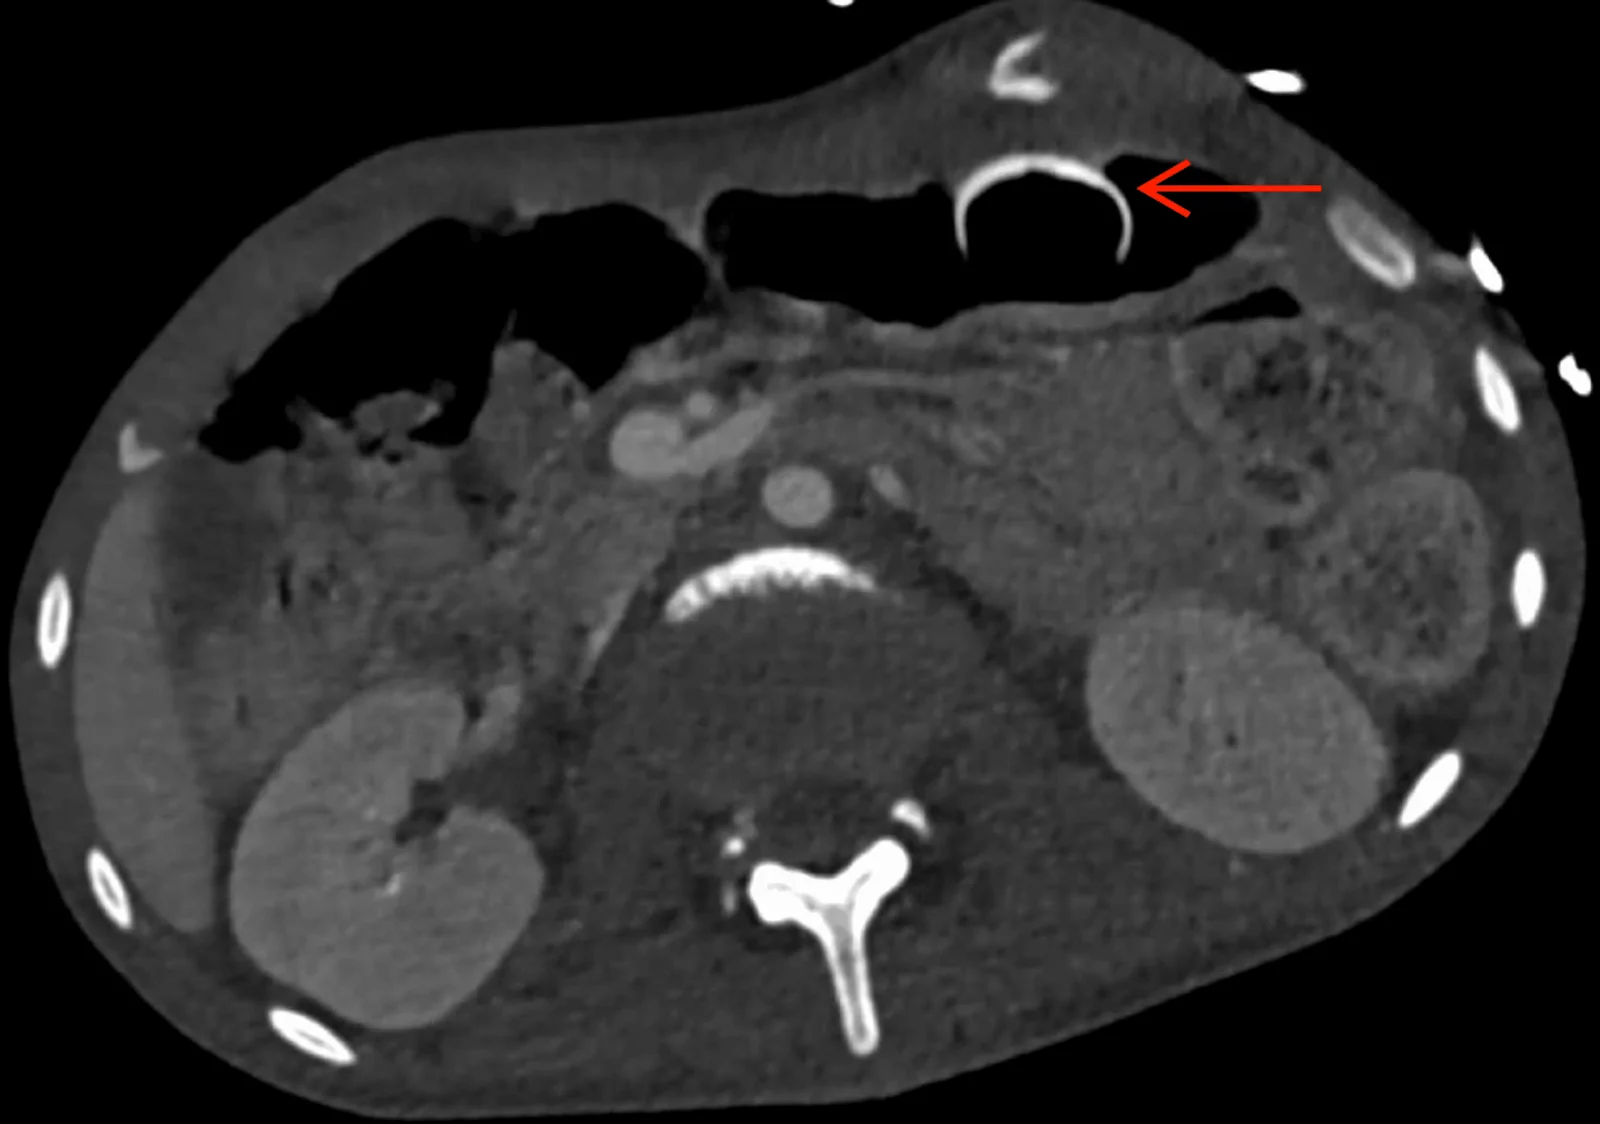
Non-contrast-enhanced CT of the abdomen and pelvis demonstrating a previously placed PEG tube within the stomach Arrow depicts percutaneous endoscopic gastrostomy (PEG) tube within the stomach.
